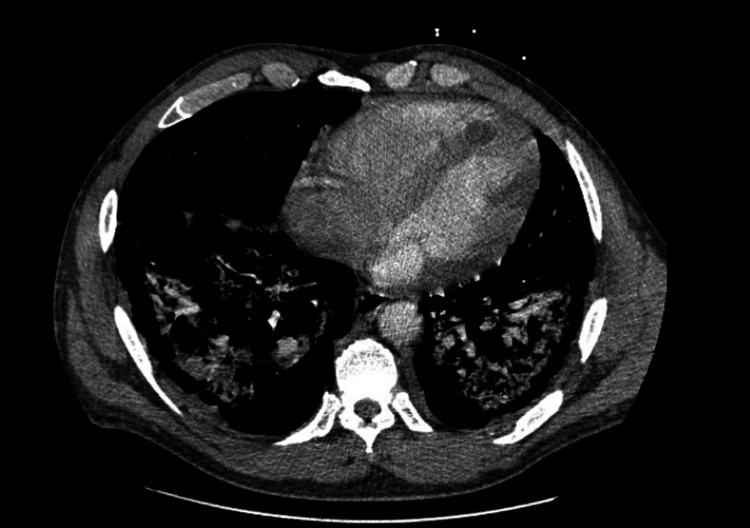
CT scan of the chest There is no CT evidence of pulmonary embolism in the proximal pulmonary arteries, although evaluation of the segmental and subsegmental arteries is limited due to bolus timing. Diffuse bilateral ground-glass opacities and consolidations have worsened since the previous exam. A dilated main pulmonary artery is observed, indicating pulmonary artery hypertension. Additionally, prominent mediastinal lymph nodes are likely reactive
